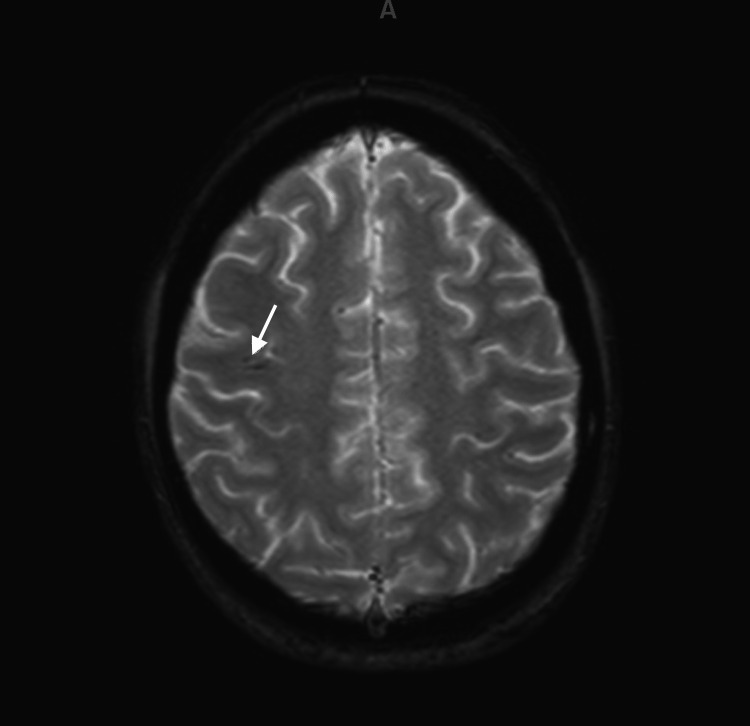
Brain MRI showing chronic cerebral hemispheric watershed infarct
